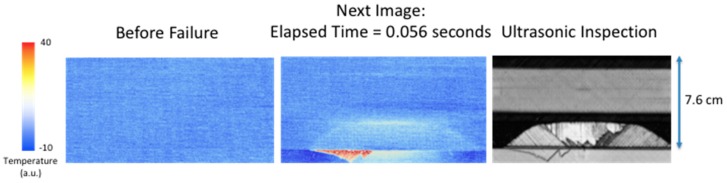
Comparison of large area instantaneous surface heating to ultrasonic inspection.
